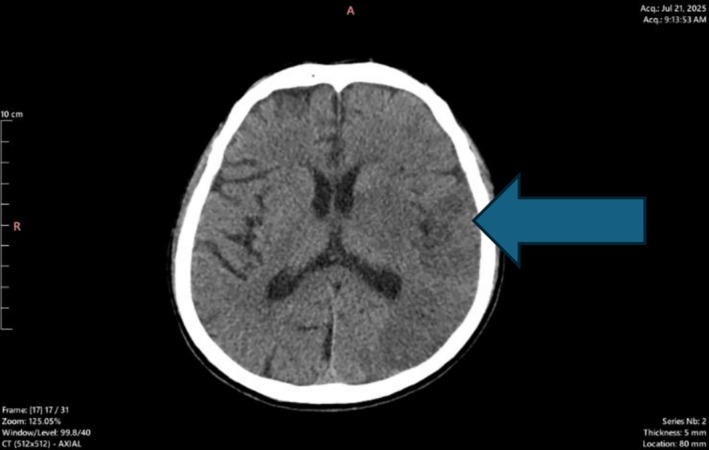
Repeat CT brain done after 15 h of thrombolysis showing left sided ischemic infarct (shown by arrow).
